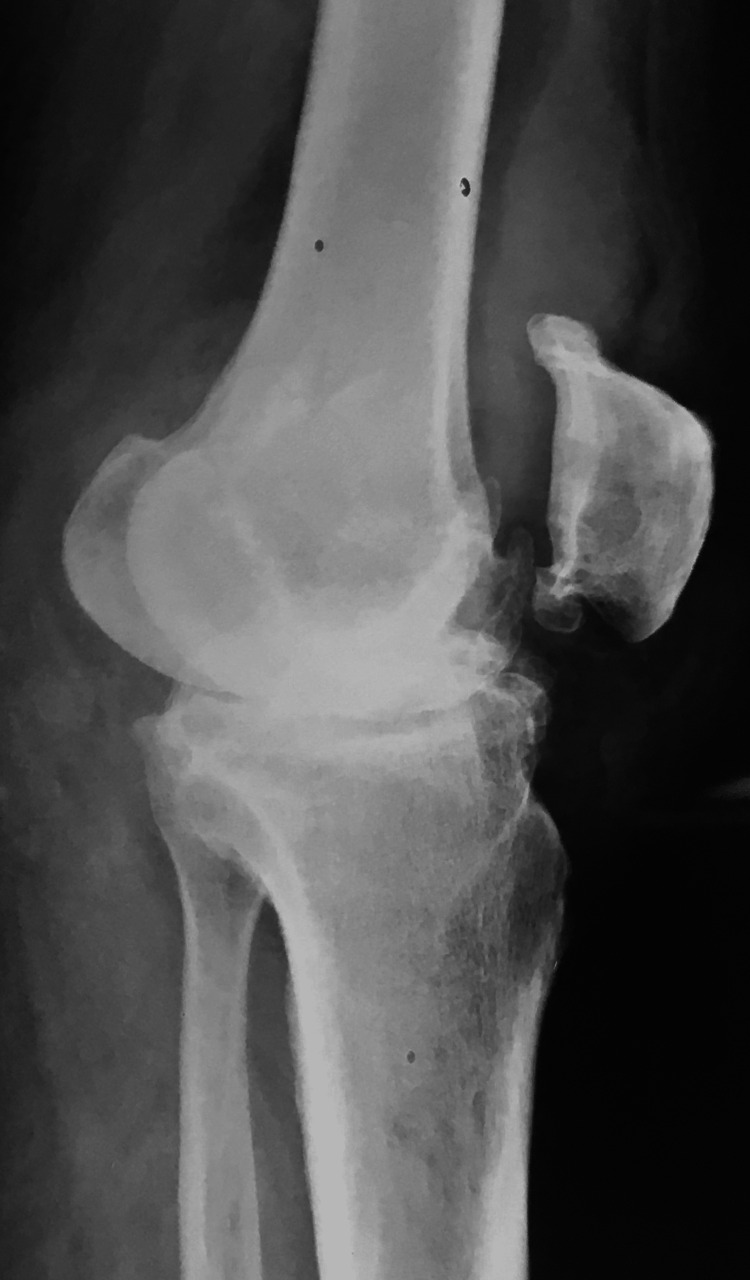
Preoperative standing lateral X-ray view For the X-ray, consent and permission were obtained from the patient.
